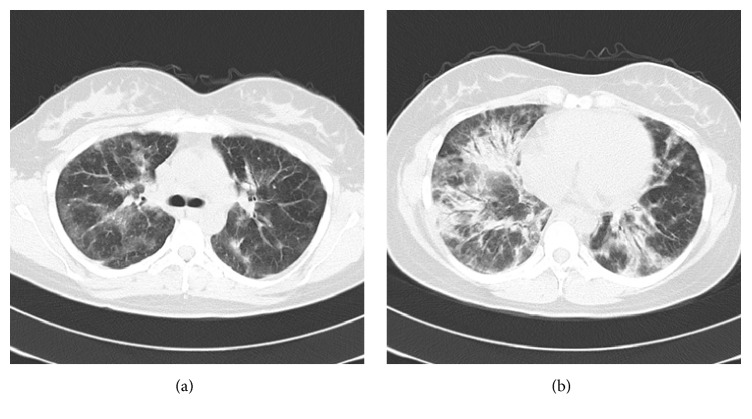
Chest CT scans showing the patient's middle (a) and lower (b) lung fields upon admission. Bilateral pulmonary infiltrates along the peripheral bronchovascular bundles and ground-glass opacities with a panlobular distribution were observed.
